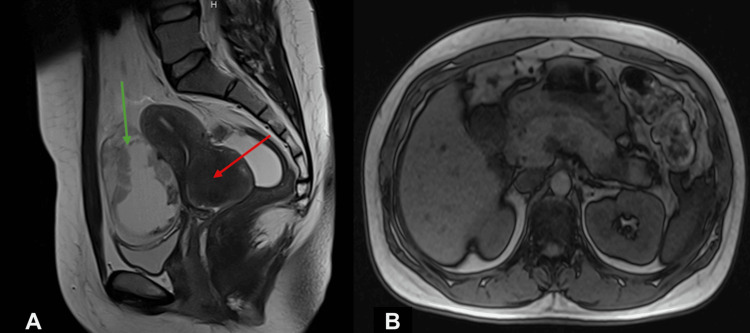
Abdominopelvic MRI (A) Abdominopelvic MRI showing a posterior-lateral cervical mass (red arrow) and concomitant, extensive, heterogenous, mainly cystic mass (green arrow) apparently arising from the left ovary; (B) abdominal MRI showing pancreatic edema with multiple nodular lesions compatible with metastasis from the extra-pancreatic site.
